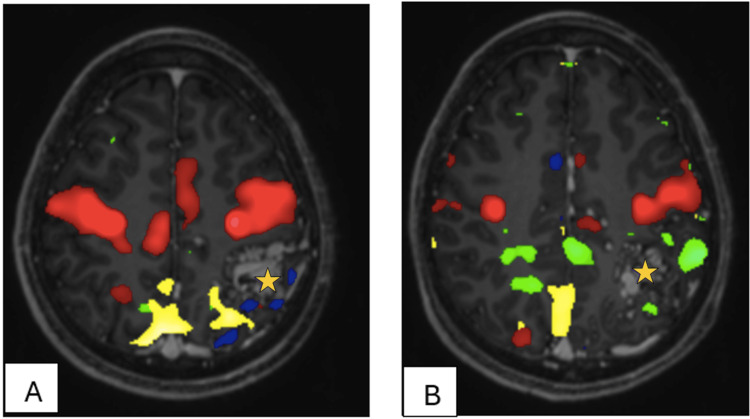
(A & B) Functional MRI of the patient while patient doing handgrip and vibrotactile stimuli of the fingertips for both hands to assess sensorimotor of bilateral upper limbs. The nidus (light orange star) is surrounded anteriorly by the motor region (red) and laterally, medially and posteriorly by the sensory region (blue, yellow and green).
